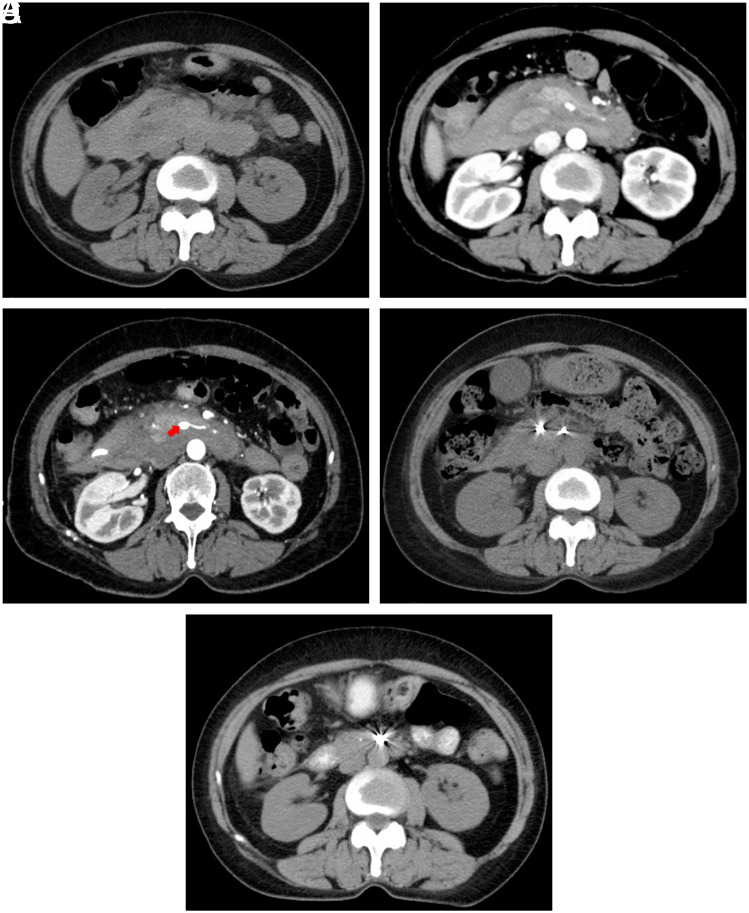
Change of this lesion on CT scans. (A) Irregularly shaped lesion around the pancreas on unenhanced CT scans. (B) Irregularly shaped and high-density retroperitoneal lesion on contrast-enhanced CT scans. (C) Enlargement of the superior mesenteric artery branch (red arrow) on CT angiography scans. (D and E) Radiation artifacts and a reduction in the size of the retroperitoneal hematoma at first and 10th weeks after endovascular treatment on unenhanced CT scans.
